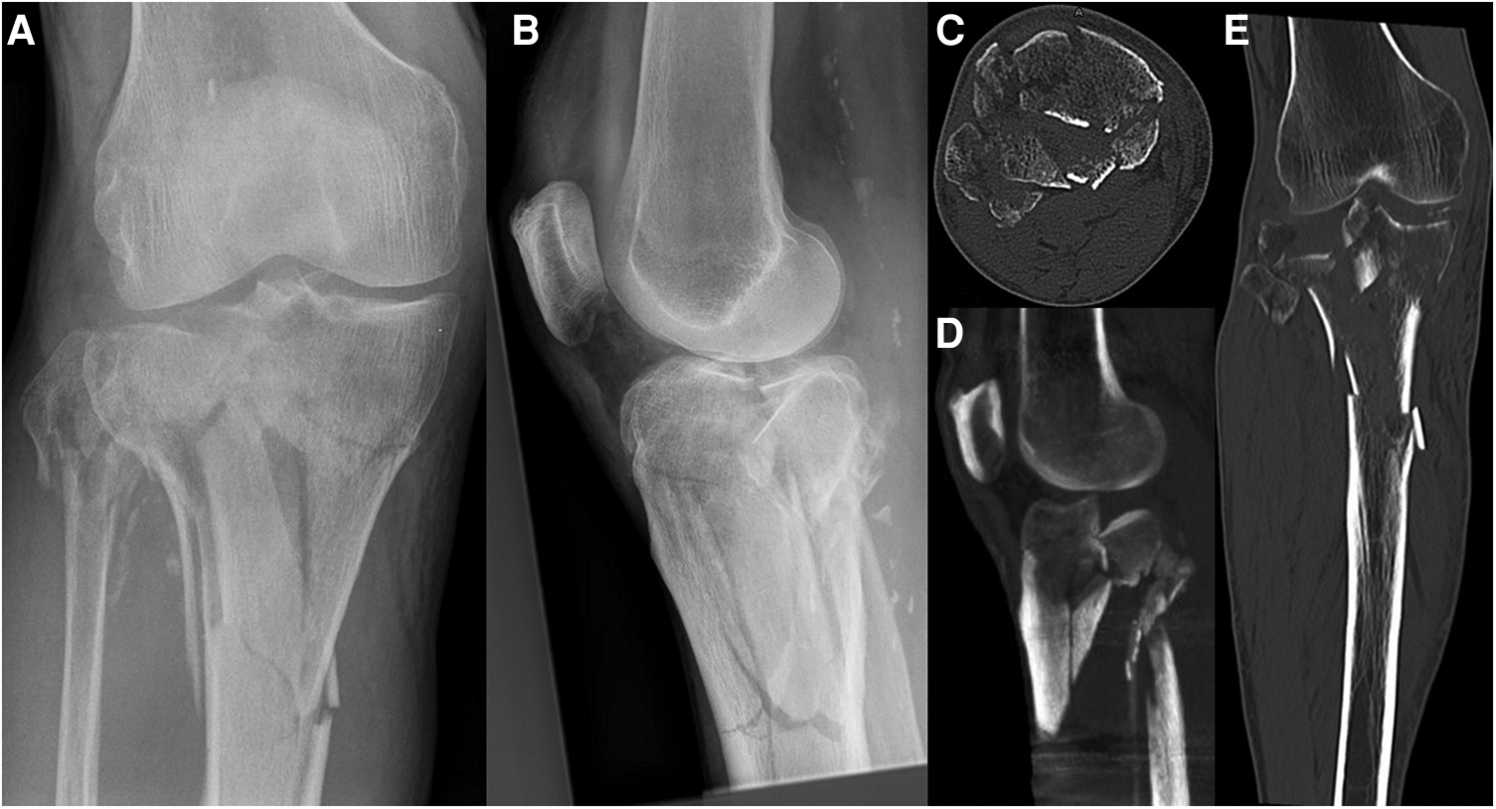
Case 3, 64 year male patient, homeless person, alcohol-related fall onto track bed. Comminuted 3-column fracture extending into diaphysis with initial valgus deformity, closed soft tissue damage grade II according to Tscherne and Oestern, uncooperative patient. AP (A) and lateral (B) view, axial CT cut (C), coronal (D) and sagittal (E) reconstruction.
